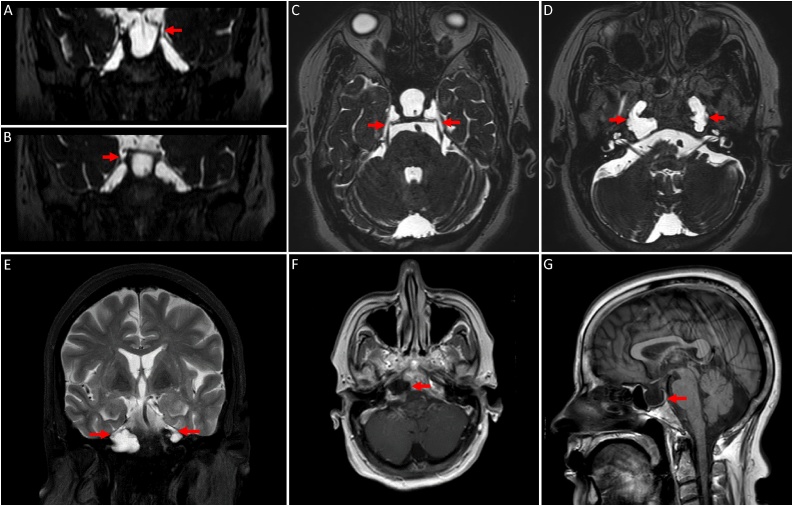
MRI of the brain; (A-D) Coronal and axial steady state sequences / FIESTA. (E) Coronal T2 weighted images / T2WI with fat suppression. (F) Axial T1WI post contrast. (G) Sagittal T1WI. (A-F) The images demonstrate bilateral, lytic, expansile, lobular lesions extending from Meckel’s caves into the petrous apices. These lesions follow CSF signal on all sequences with no enhancement. (G) An associated empty sella is noted.
